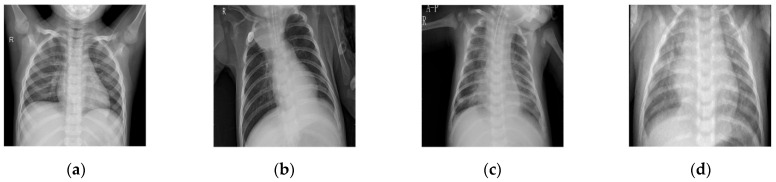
COVID-19 reference CT image dataset; (a) CT1 image; (b) CT2 image; (c) CT3 image; (d) CT4 image.
